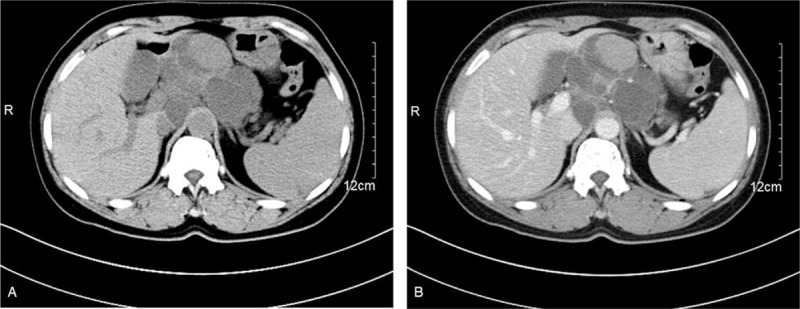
Abdominal CT images revealing a retroperitoneal cystic and solid mass located near the midline. (A) Axial nonenhanced CT image showing that the mass was mainly multiseptated in the cystic portion and had a bead-like, lobulated appearance. (B) Axial contrast-enhanced CT image showing a solid portion with obvious homogeneous enhancement from 42 HU to 73 HU and a cystic portion with ring-like and septal enhancement. CT = computed tomography, HU = Hounsfield units.
